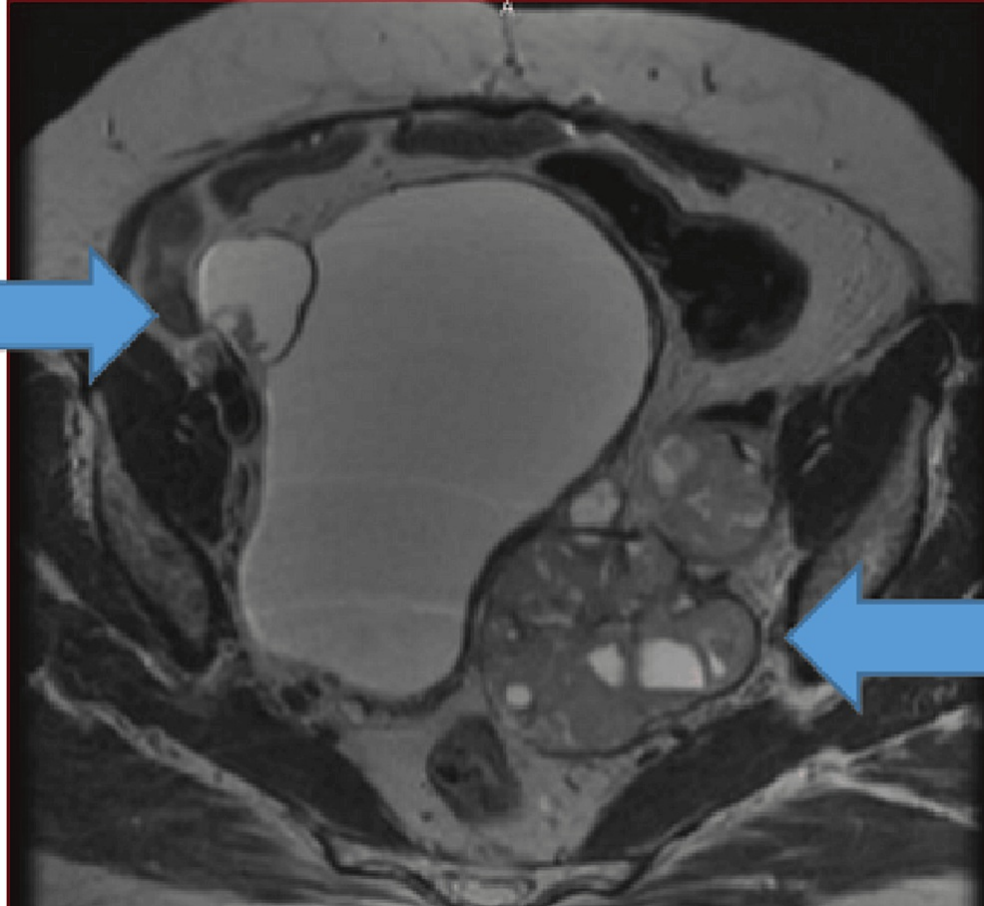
MRI of the pelvis T2 sequence axial view showing interval progression of the large multilobulated left adnexal mass and new right adnexal complex masses, suggesting disease progression.
